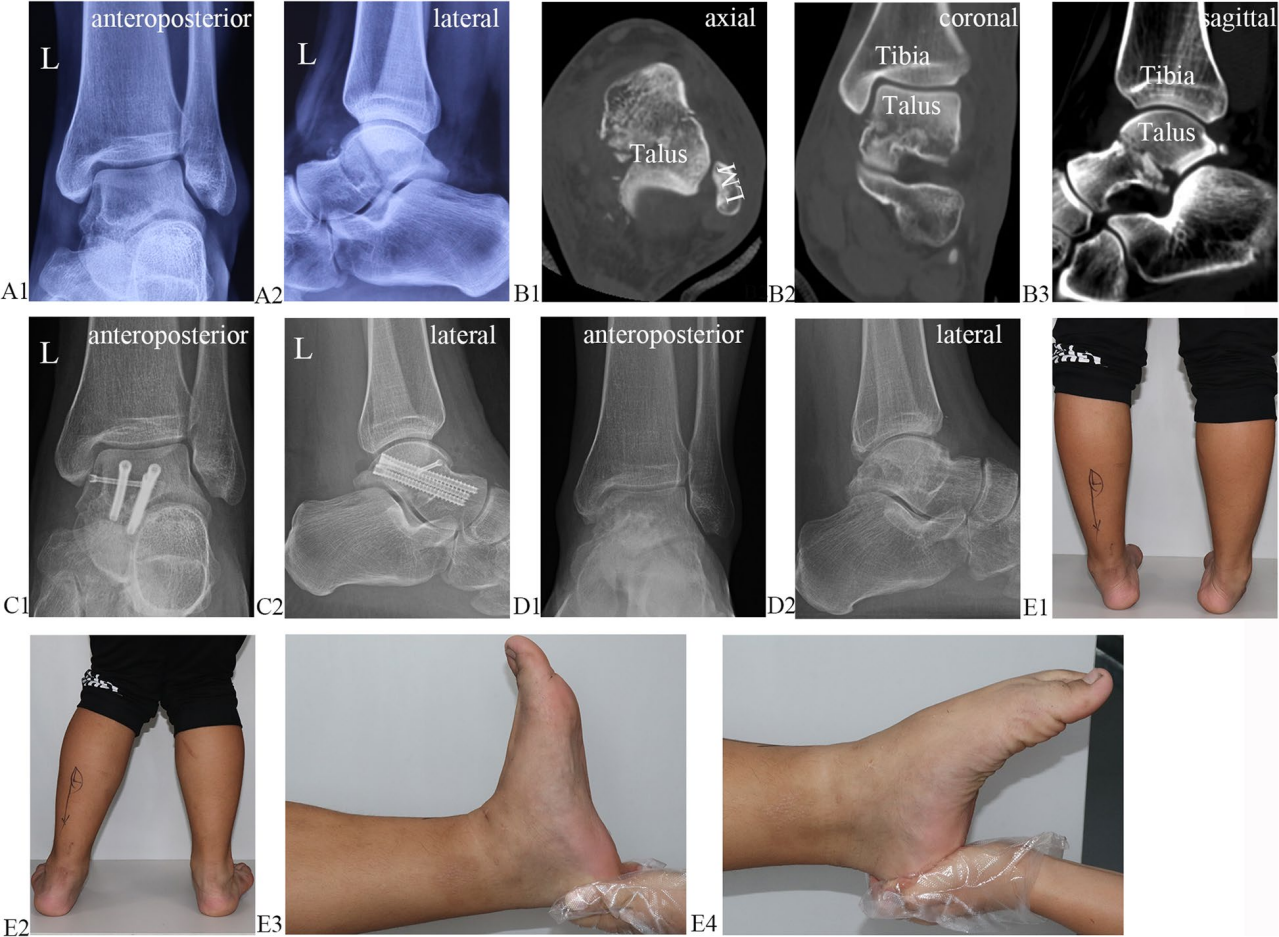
Preoperative X-ray radiography (A1 and A2) and CT (B1–B3) results. X-ray radiography results one week (C1–C2) and two years (D1–D2) after the operation. Functional results two years after the operation (E1–E4)
